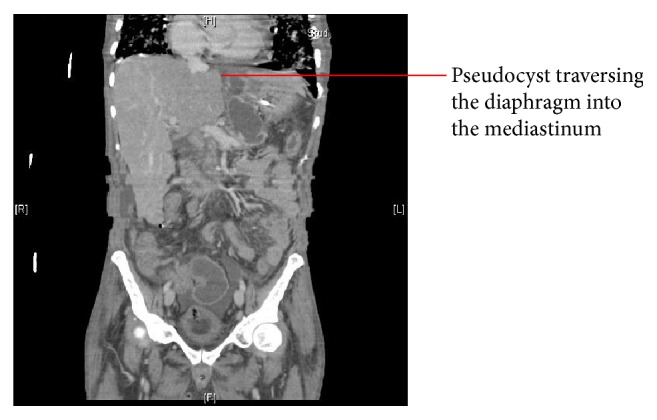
Abdominal CT showing cyst extension into mediastinum.
